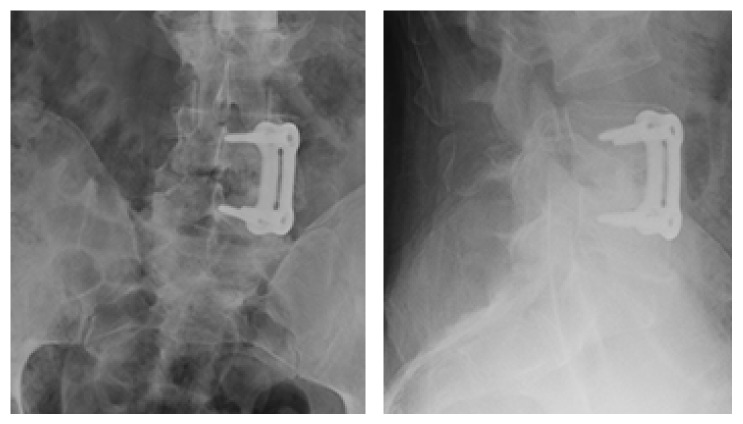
AP and lateral radiographs of lower lumber spine showing the anterolateral locking plate at L4-5 (Synthes 4.5 mm anterolateral spinal locking plate, with 5.5 mm titanium locking screws, West Chester, Pennsylvania, United States).
